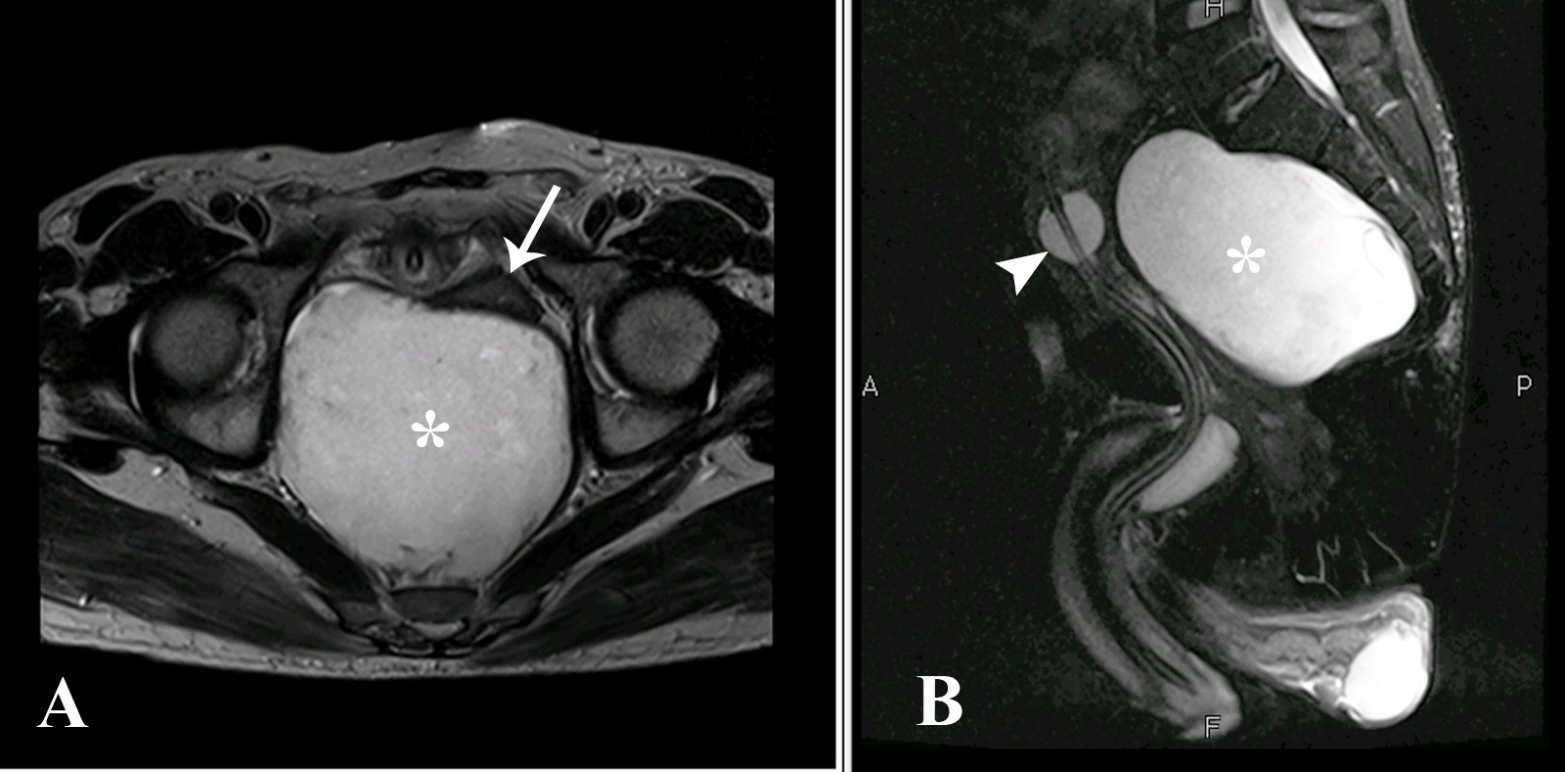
Axial (A) and sagittal (B) T2-weighted MR sequences The cystic lesion (asterisk) is hyperintense on T2 weighted imaging, with few foci of T2 hypointensities in the periphery correlating with keratin. It is compressing the rectum anteriorly (arrow) and the urinary bladder which contains a Foley's catheter in situ (arrowhead). MR: magnetic resonance
